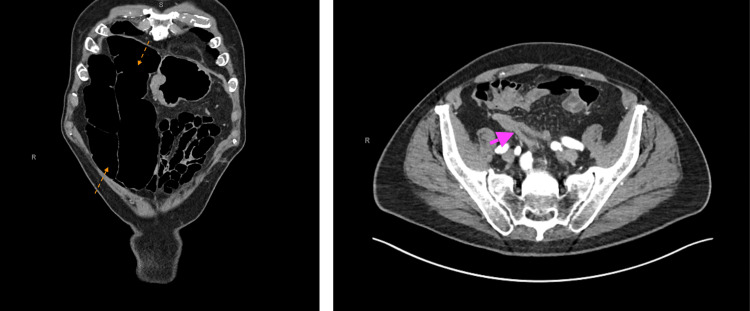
On the coronal view (left), CT imaging of the abdomen revealed a large volvulus, while the axial view (right) reveals the transition point at the sigmoid volvulus. Orange arrows depict the dilated bowel loops caused by the volvulus. The pink arrow depicts the transition point at the sigmoid volvulus.
